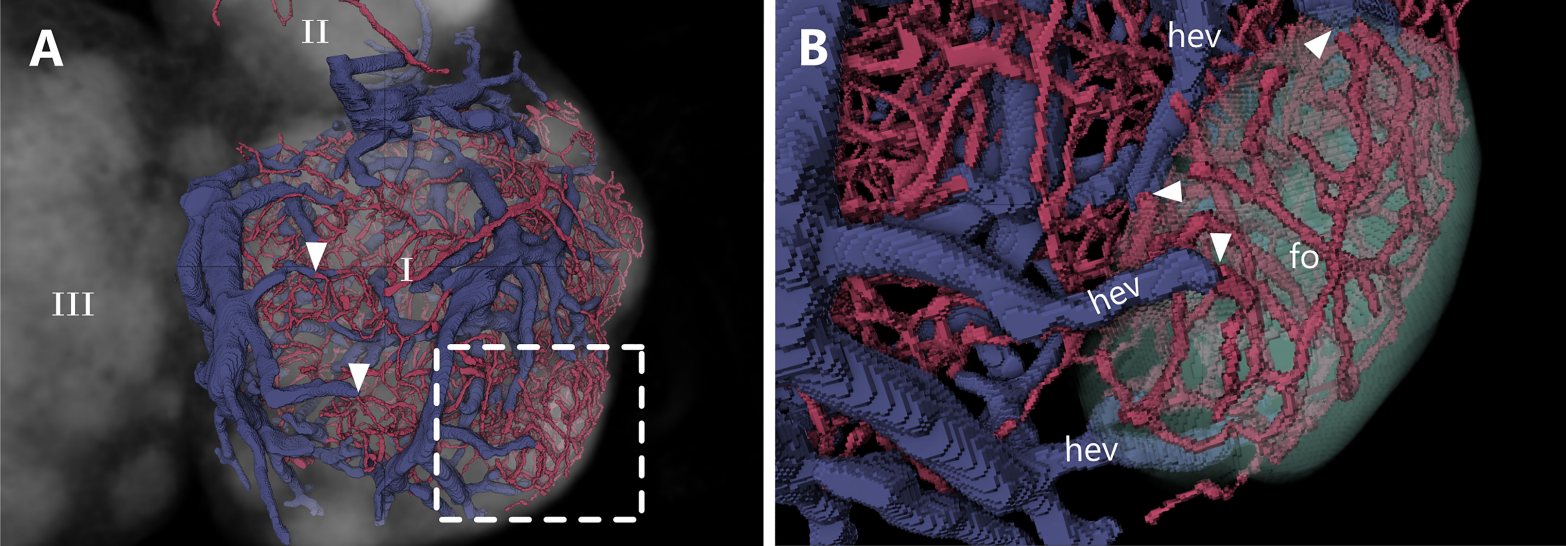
Transition from capillary beds to high endothelial venules (HEVs) at the B-cell follicle margin. An animated visualization of this figure is available in the supplementary files (Supplement Video S5). (A, B) Volume rendering of an image stack acquired by means of X-ray phase-contrast imaging with a 2D Talbot array. The lymph node is displayed in a top view. (A) Capillarization and venule distribution of one lobe (I). Transitions of capillaries into HEVs are clearly visible due to an abrupt change in vessel diameter with a ratio of 1:3 (arrowheads). Greater magnification in panel B (dashed line). (B) Close up shows a dense capillary bed within a B-cell follicle which gets drained by multiple HEVs. HEVs are closely located at the margin of the follicle. B-cell follicles=fo (mint green), high endothelial venules=hev (blue).
